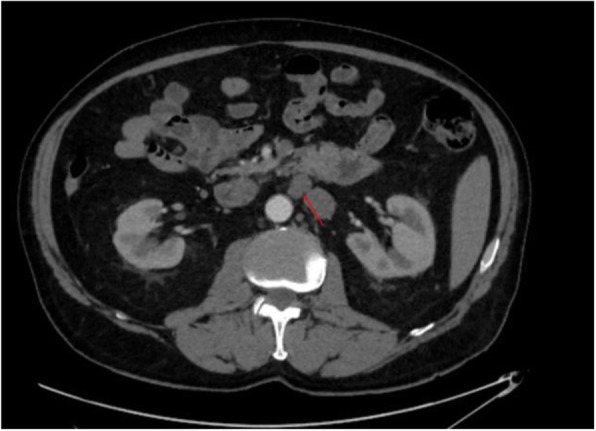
Enlarged to 21 × 15 mm infrarenal paraaortic masses in computed tomography axial view
